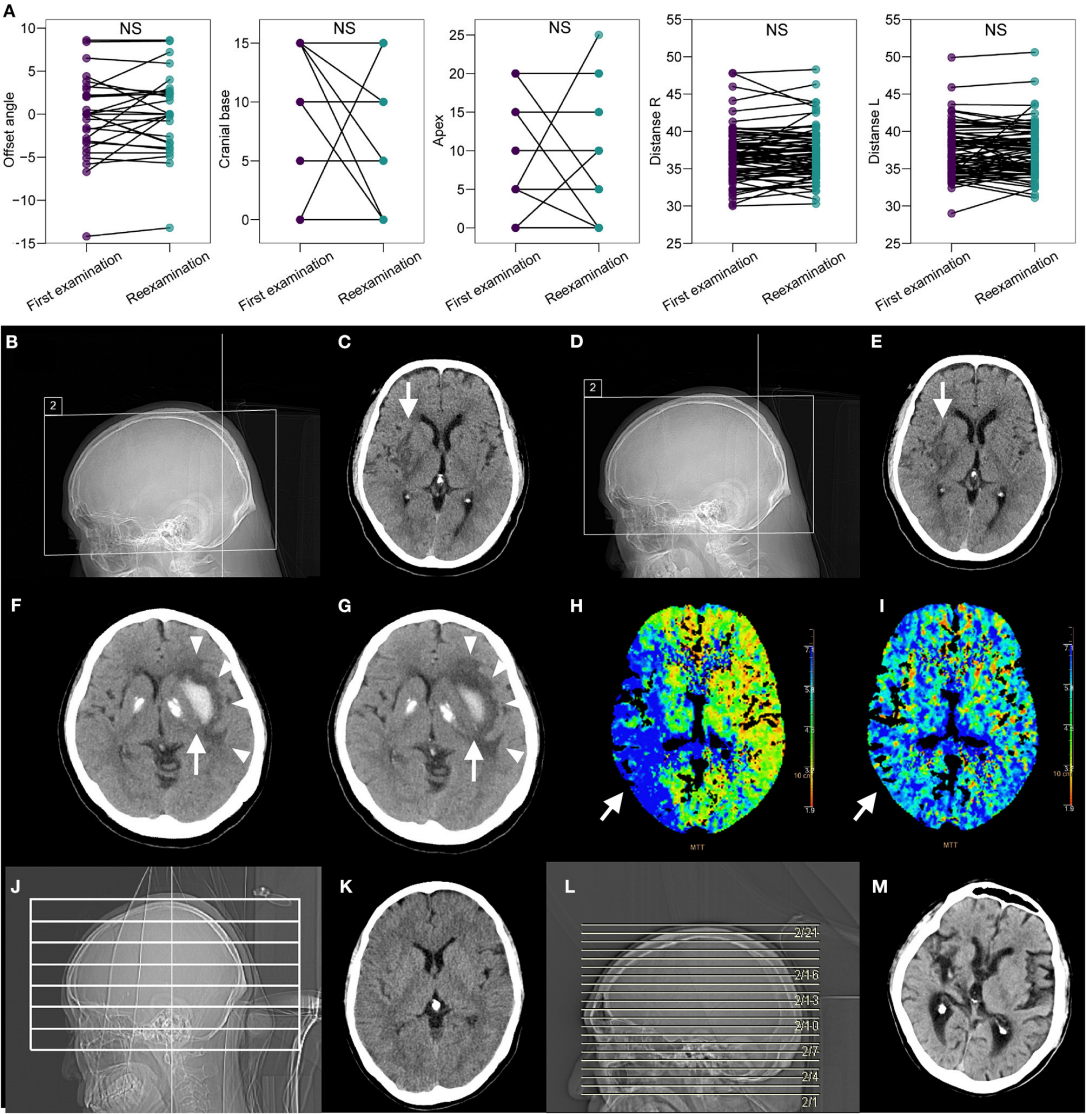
Repeatability of CAPITAL-CT compared with the repeatability of traditional CT. (A) Pairwise comparison revealed no significant difference between the first examination session and the reexamination session regarding offset angle, apex edge, cranial base edge, distance from both lateral ventricles to the intracranial plate, and the distance between bilateral ventricles (all paired *t*-test *P* > 0.05 and labels as ns for non-significance) for images collected from a total of 77 patients who required reexamination. (B–E) The patient diagnosed with ischemic cerebral infarction in the right basal ganglia (white arrow) has an ASPECTS score of 7 points. The score was reassessed as 7 points upon reexamination because the images obtained from the first examination and reexamination almost completely overlapped. (F,G) In the patient with cerebral infarction in the area dominated by the right middle cerebral artery (white arrow), the comparison of the mean transit time (MTT) images before and after thrombus recanalization showed that the levels were consistent, and the MTT before and after the thrombus had obvious improvement in blood flow. (H,I) For the patient with hemorrhagic stroke in the left basal ganglia area, the white hematoma (white arrow) was found to be significantly reduced, but the surrounding edema (white triangles) did not change after a few days of reexamination, and the images of the two sequences completely overlapped. (J–M) Compared with other traditional CT imaging results, CAPITAL-CT-derived images are highly reproducible.
